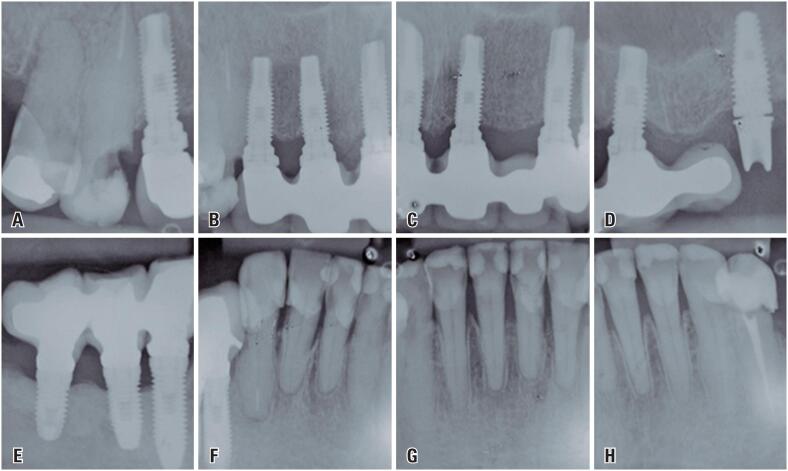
Initial radiographic images. Presence of prosthesis over implant in region of 12, bone loss in distal of tooth 14 and, radiolucent area in mesial of tooth 13, which was endodontically treated (A); Presence of prostheses over implants from 12 to 25, and 44 to 46, with normal bone pattern (B, C, D and E); Implant associated with its prosthetic component in region of 26, but with loss of the prosthetic crown (D); Teeth from 34 to 43, extensively restored (F, G and H), and 34 was endodontically treated and restored with prosthetic crown (H)
